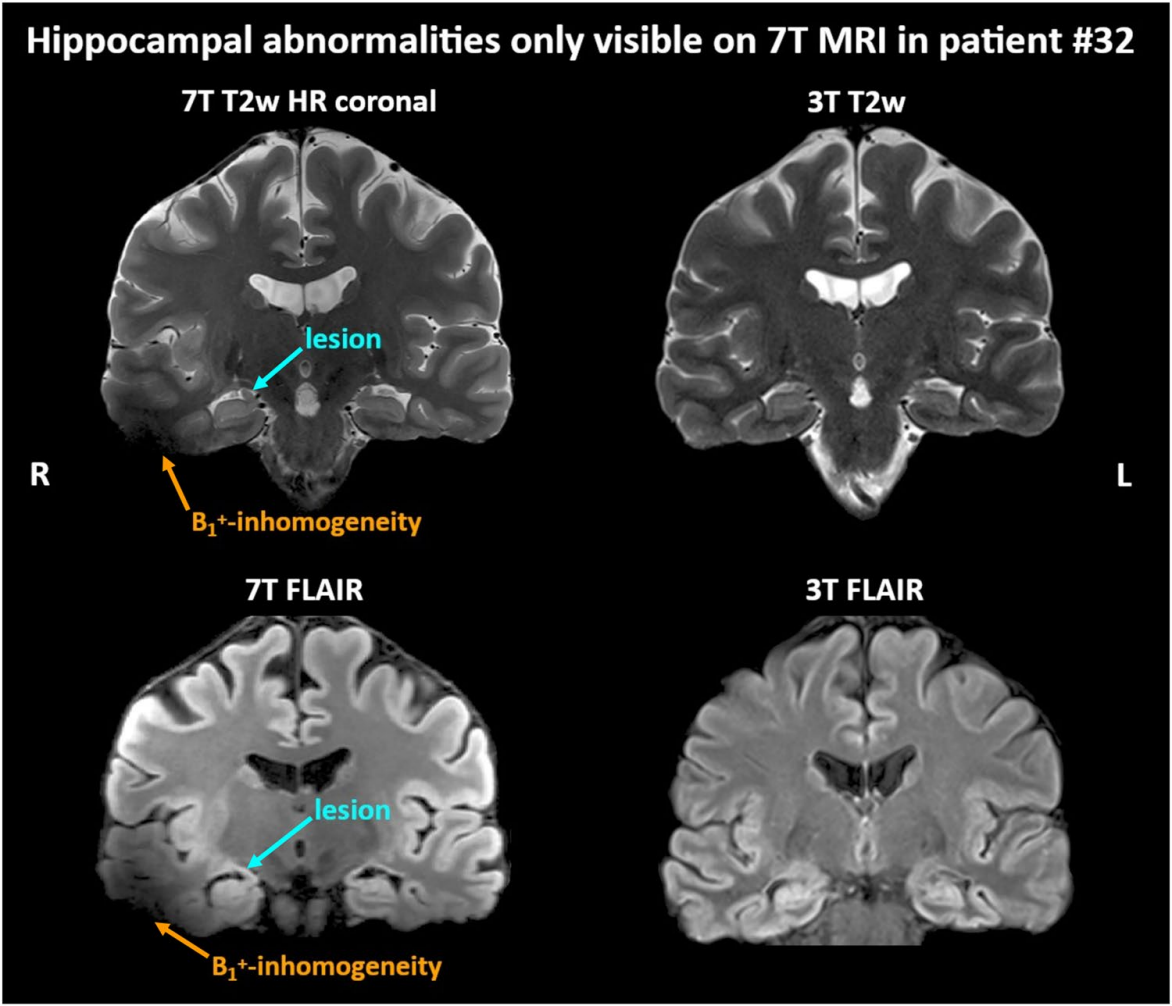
In this case, patient #32, 7 T MRI could identify hippocampal asymmetry regarding size and structure of the right hippocampus whereas 3 T MRI remained negative. On T2w 7 T MRI, the right hippocampus appeared larger during neuroradiological evaluation than the left side. Arrows highlight regions of interest for pathology or artifacts. *T2w*  T2-weighted, *FLAIR*  Fluid-attenuated inversion recovery, *7 T*  7 Tesla, *3 T*  3 Tesla, *HR*  high resolution, *B1*^+^  radio frequency excitation, *R* right side, *L* left side
